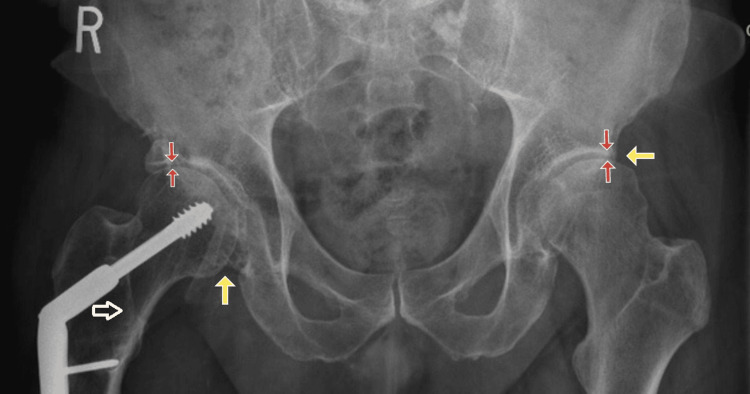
Bilateral hip X-ray The X-ray imaging shows postoperative changes of the right femur with bilateral acetabular dysplasia (yellow arrows), a healed right intertrochanteric stress fracture (white arrow), and progression of bilateral coxarthrosis (red arrows).
